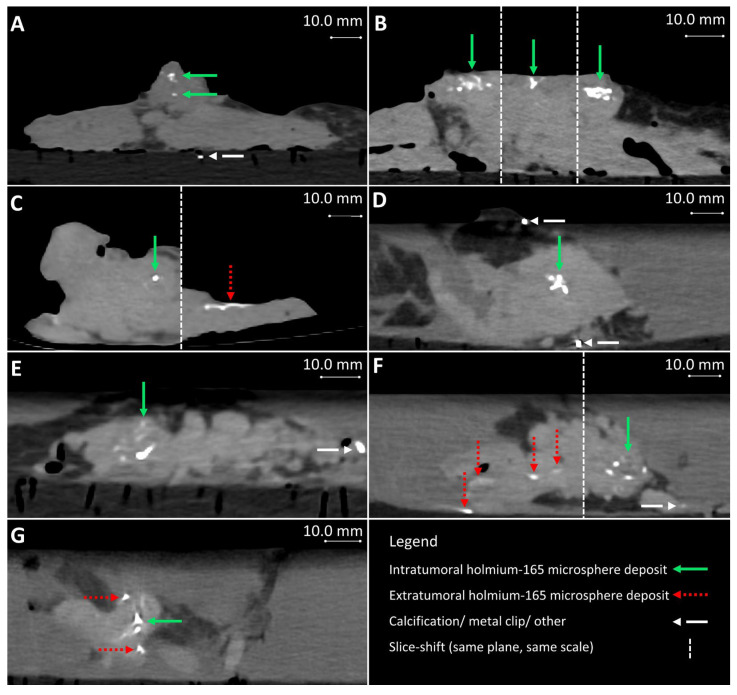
Post-injection CT from samples 4–10 (A–G), respectively. (B,C,F) contain multiple slices of the same plane and scale. Extratumoral holmium-165 microsphere deposits occurred and are visible in images (C,F,G), for samples 6, 9, and 10, respectively.
